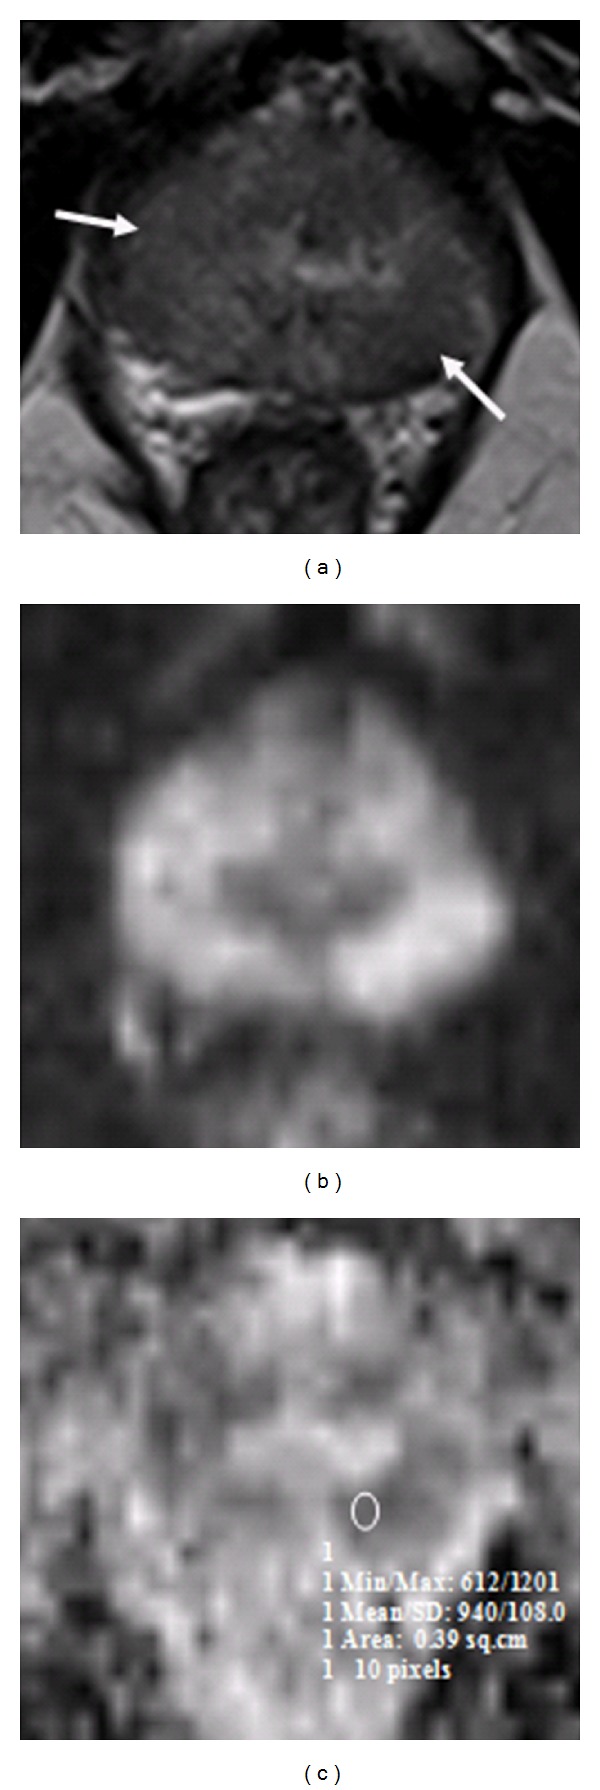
Patient with low-risk prostate cancer. Pelvic T2W transverse image (a) shows a well-defined low signal intensity region in the peripheral zone on the right and left mid zones (arrows). (b) DWI-hyper intense signal in corresponding areas. (c) A radiologist-determined region of interest on ADC map shows restricted diffusion in right and left peripheral zones, scored as Gleason 6 on sextant biopsy.
